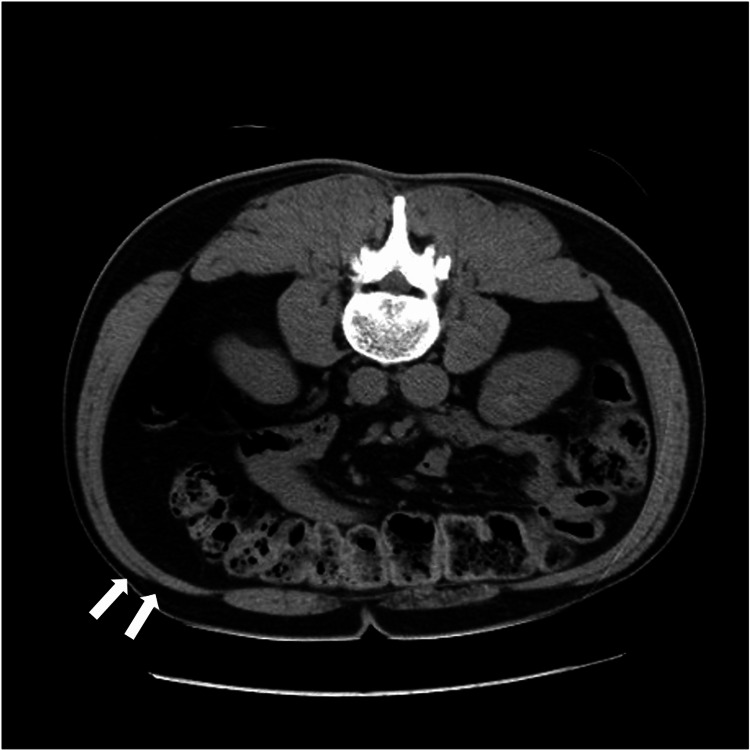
Computed tomography image. A mass or hernia was not detected, and the patient’s diagnosis was unclear. However, a CT scan reveals retrospectively slight muscle atrophy at the right flank compared with the left flank (arrow).
